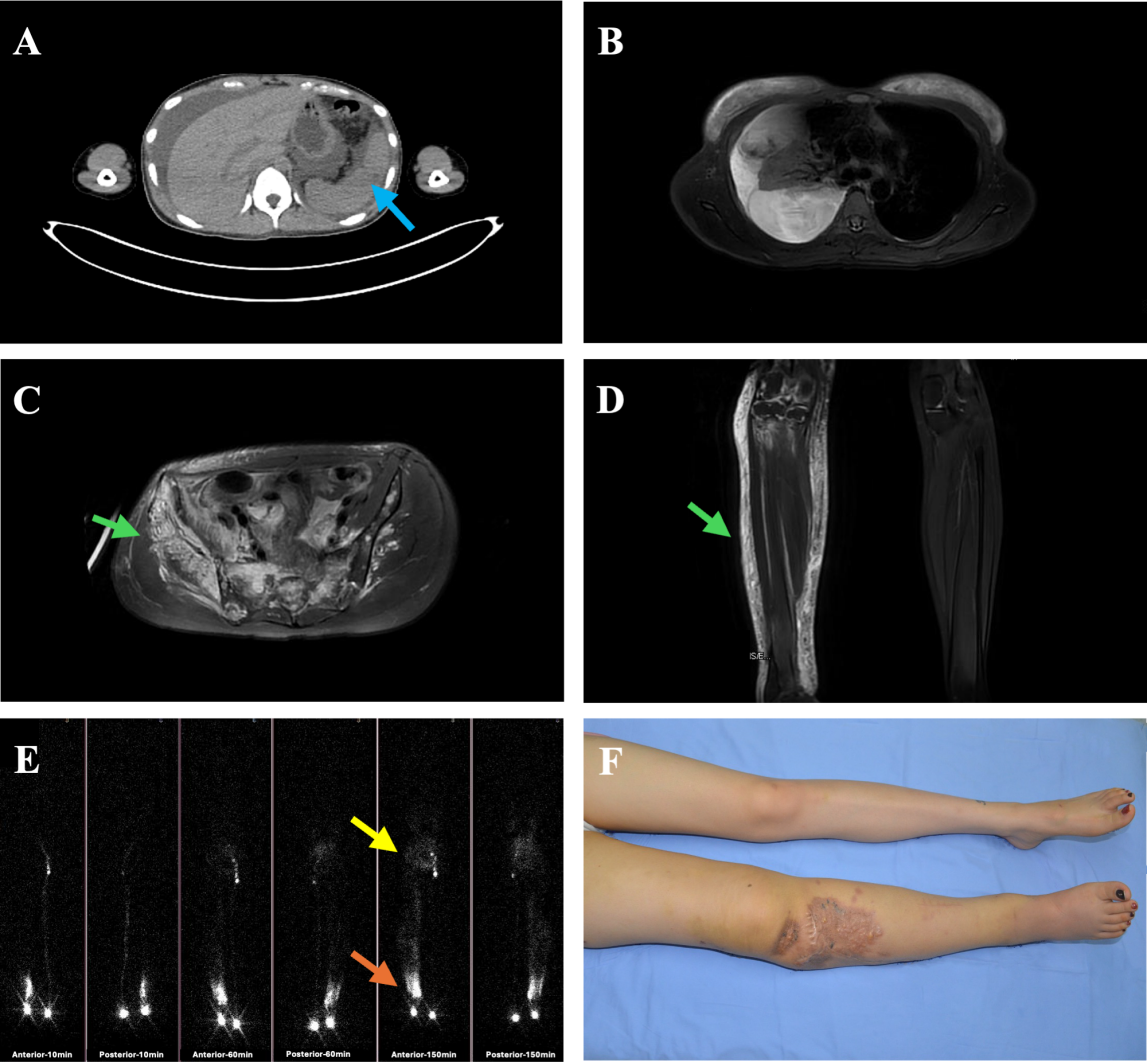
A female patient with Generalized Lymphatic Anomaly (GLA) manifested pleural and peritoneal effusions, cystic lesions of the spleen, and right lower extremity lymphedema. (A) Peritoneal effusion and nodular lesions in the splenic parenchyma are visualized on computed tomography (CT) (blue arrows). (B) Massive right-sided pleural effusion with significant compression of the right lung is evident on axial T2-weighted MRI. (C, D) High-signal-intensity lesions involving the dermis, subcutaneous tissue, and deep fascia of the right inguinal region and lower extremity are demonstrated on axial and coronal T2-weighted images, accompanied by perilesional soft tissue edema (green arrows). (E) Lymphoscintigraphy reveals non-visualization of right inguinal lymph nodes at 10, 60 and 150 minutes post-injection in the right foot (yellow arrows), along with abnormal tracer accumulation and cutaneous reflux in the right lower extremity (focal tracer retention highlighted by orange arrows). (F) Extensive right lower extremity swelling is documented in the clinical photograph.
